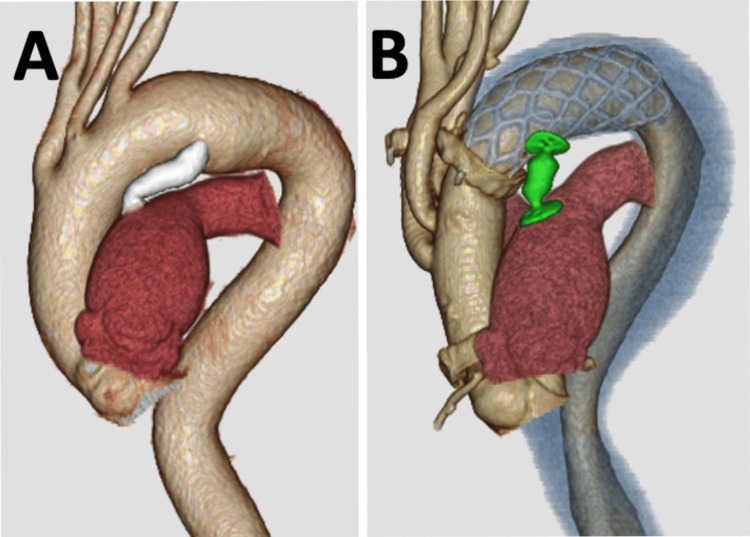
3D computed tomography scan Before the procedure, a large PDA was shown by computed tomography scan (A). Postoperative computed tomography scan revealed successful coverage of the intimal tear and thrombosis in the false lumen (B). (Green device: AVPII; Colored blue lumen: Thrombosis in false lumen) PDA: Patent ductus arteriosus; AVPII: Amplatzer Vascular Plug II
